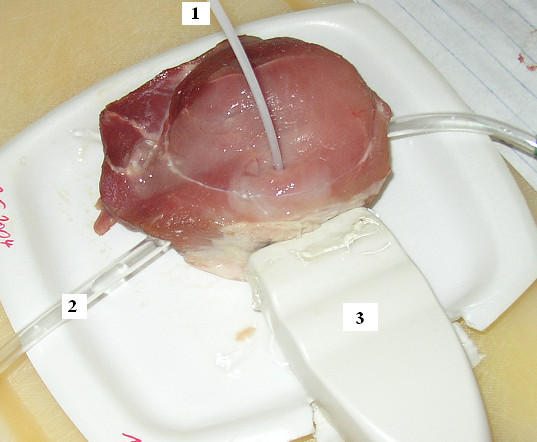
The experiment setup. 1- laser applicator, 2- transparent tube (considered as a blood vessel), and 3- is ultrasound sonography prob. The tube was introduces by pulling it through a hole made using dipped knife. The online sonography was used to verify the position of the applicator.
